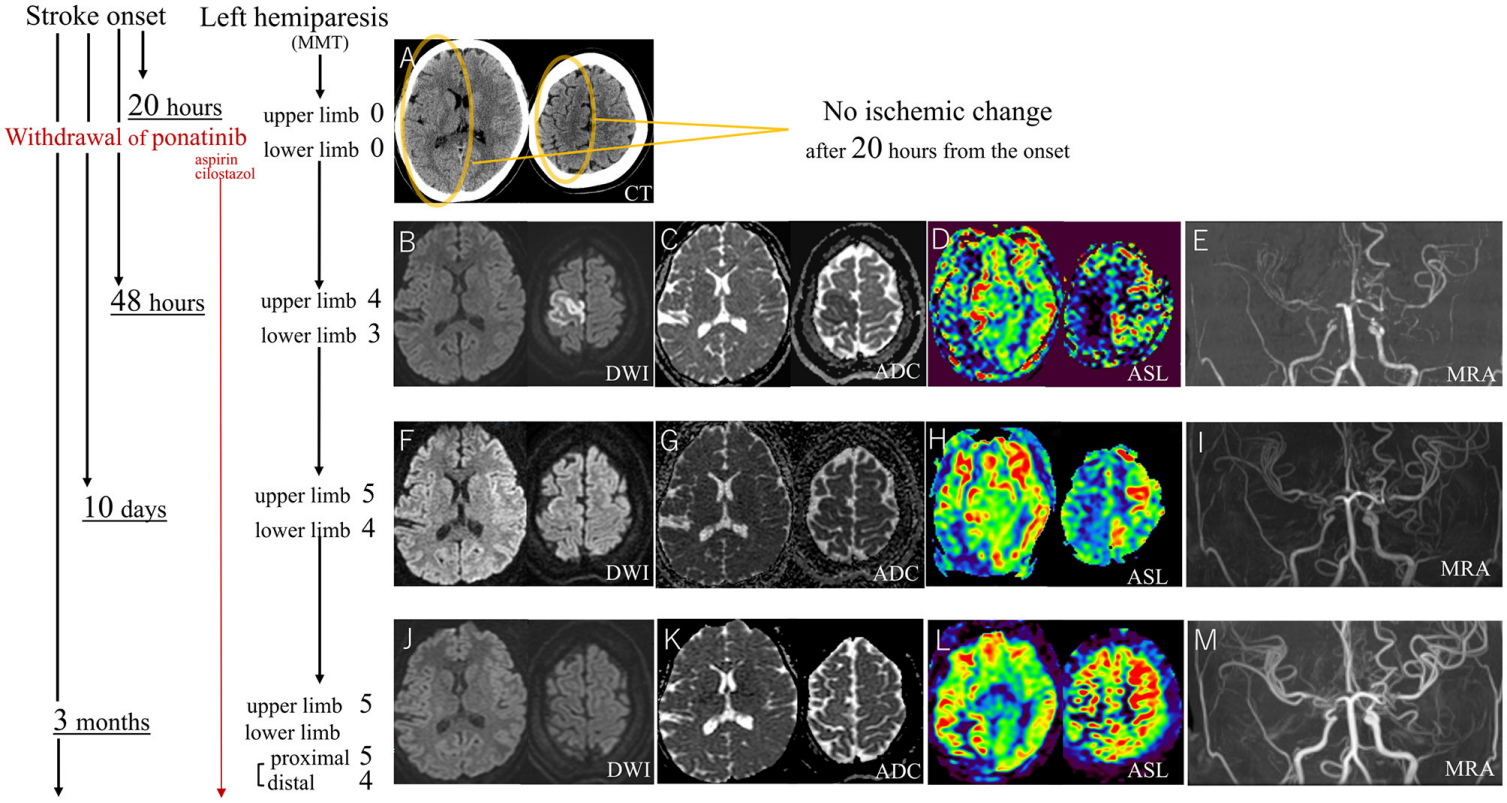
After 20 h of stroke onset with severe MMT-0 left hemiplegia, the CT scan shows no ischemic changes (A). DWI and ADC on MRI at 48 h from the onset show gray matter-confined infarction at the right ACA area (B, C) which is an atypical lesion in ICA stenosis (E). Ten days after the stroke onset, the MRI shows the almost disappeared ischemic lesions (F, G), and after 3 months, these lesions completely improved (J, K), ASL and MRA show striking improvement after the withdrawal of ponatinib (D, E, H, I, L, M). MMT, manual muscle test; CT, computed tomography; DWI, diffusion weighted imaging; ADC, apparent diffusion coefficient; MRI, magnetic resonance imaging; ACA, anterior cerebral artery; ICA, internal carotid artery; ASL, arterial spin labeling; MRA, magnetic resonance angiography.
